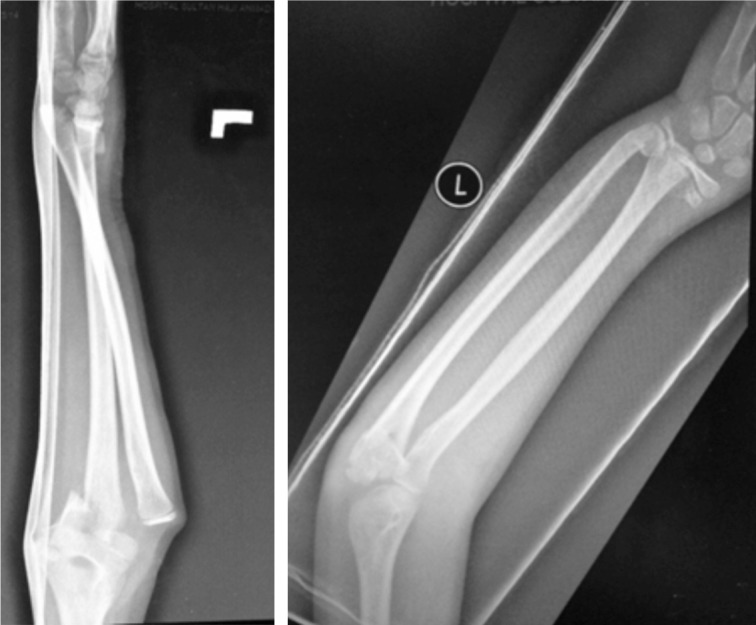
The initial radiograph taken in Emergency Department showing distal end radius Salter-Harris type II fracture with distal third metaphyseal ulna fracture with ipsilateral proximal ulna fracture with radial head posterior dislocation.
